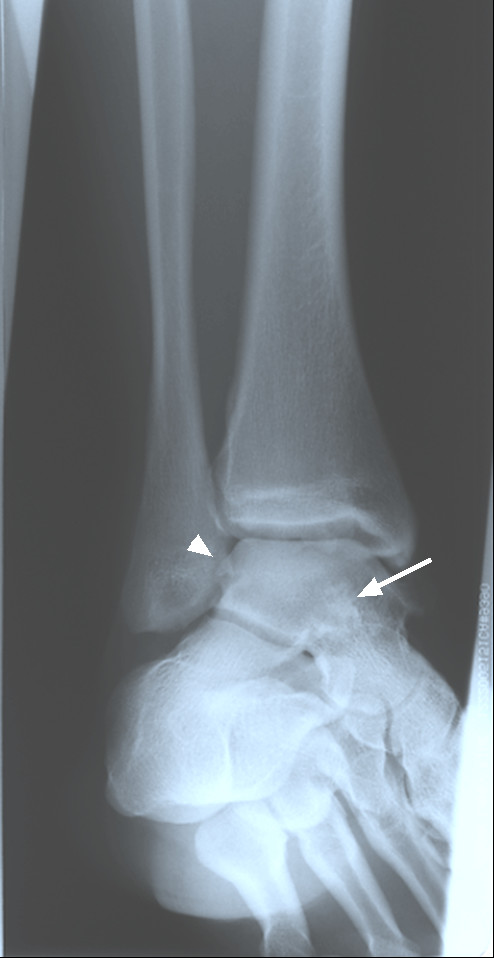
Oblique left ankle radiograph with evidence of calcified loose bodies medial (arrow head) to the lateral maleolus and superimposed over the talus (arrow). This suggests synovial chondromatosis, likely located in both the flexor hallucis and tibialis posterior tendons.
